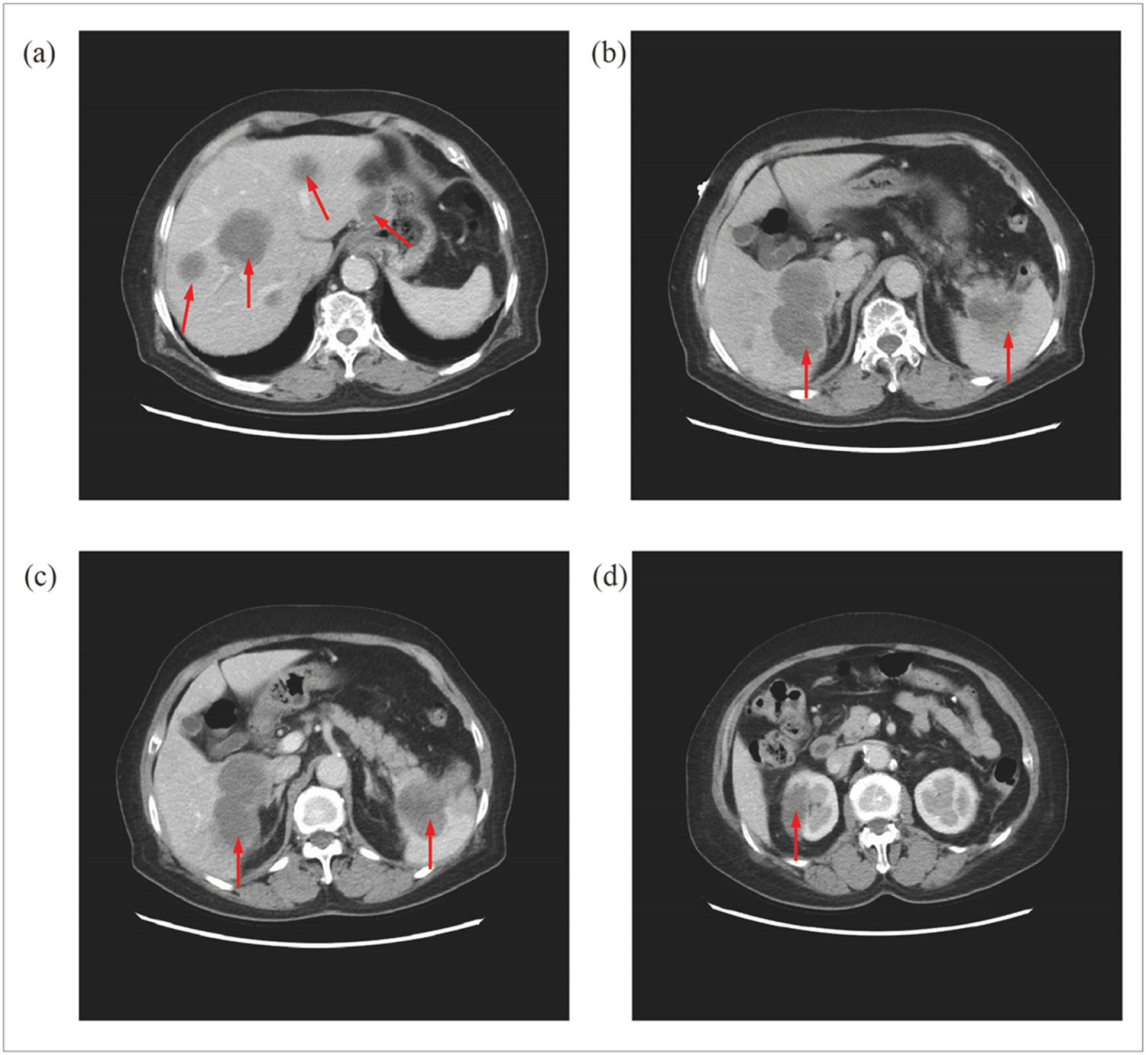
Abdominal enhanced CT. (a and b) Multiple cancer metastases in the liver and spleen. (c) Space occupying lesion of the pancreatic tail and the metastases in the liver. (d) Abnormal intensification in the right kidney.
